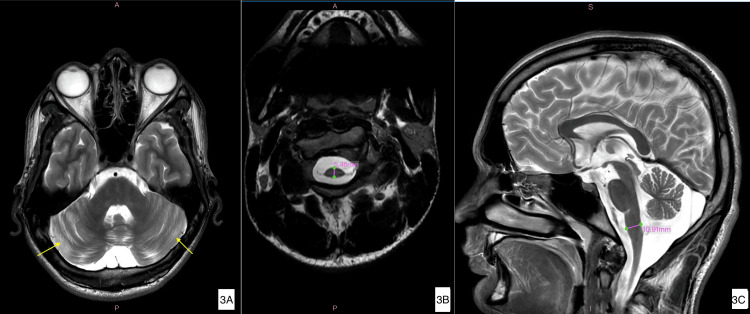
Magnetic resonance imaging (MRI) of the spinal cord and brain 3A. T2-weighted axial MRI of the brain showing prominent cerebellar folia (yellow arrows) suggestive of mild cerebellar atrophy. 3B. T2-weighted axial MRI of the cervical spinal cord (C3 level) showing reduced anteroposterior diameter of 5.46 mm, suggestive of cervical cord atrophy. (measuring marker (purple color); normal reference 8.0 mm). 3C. T2-weighted sagittal MRI brain highlighting mild medullary atrophy with an antero-posterior diameter of 10.91 mm (measuring marker (purple color); normal reference 13 mm)
